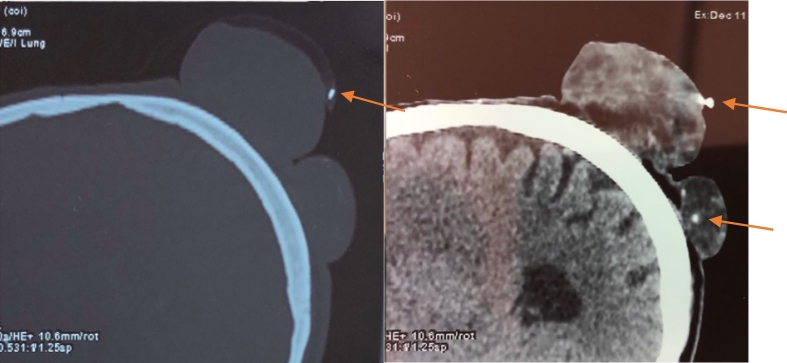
Coronal CT scan of the head showing the soft-tissue density lesions. Note the calcifications foci (arrows).
